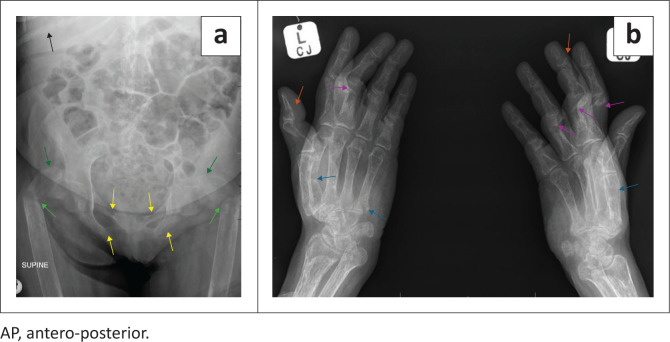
(a) AP radiograph of the pelvis: Generalised loss of bone mineral density. Cranially displaced subcapital as well as transverse sub trochanteric femur fractures (green arrows). Bilateral superior and inferior pubic rami fractures (yellow arrows). Right posterior 10th rib fracture (black arrow). (b) Oblique radiographs of bilateral hands: Generalised loss of bone mineral density. Multiple bilateral proximal phalanx fractures with dorsal angulation (purple arrows). Bilateral distal phalanx fractures (orange arrows). Bilateral metacarpal fractures (blue arrows).
